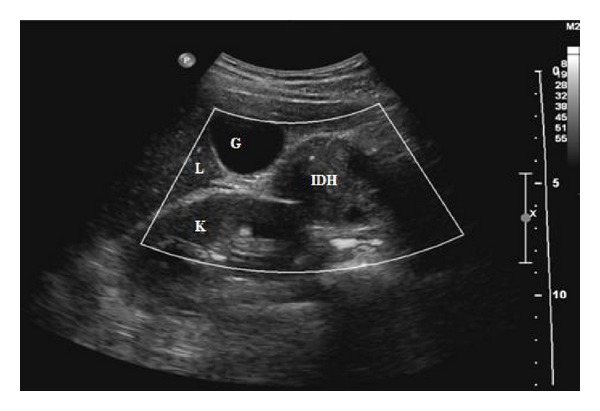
Ultrasound of the abdomen demonstrates a well-defined heterogenous 3.5 × 3 cm lesion anterior to the right kidney in the location of second part of duodenum. IDH: intramural duodenal hematoma; L: liver; G: gall bladder; K: kidney.
